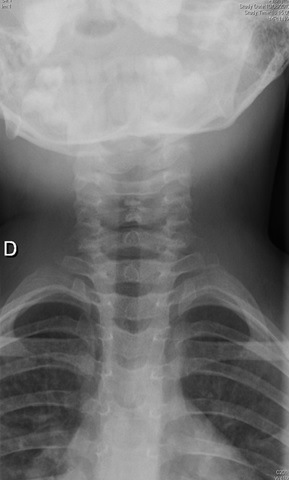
Radiographie du rachis cervical incidence de Face ou l’on voit au niveau des vertèbres C2C3 des calcifications discales étagées des disques intervertébraux
